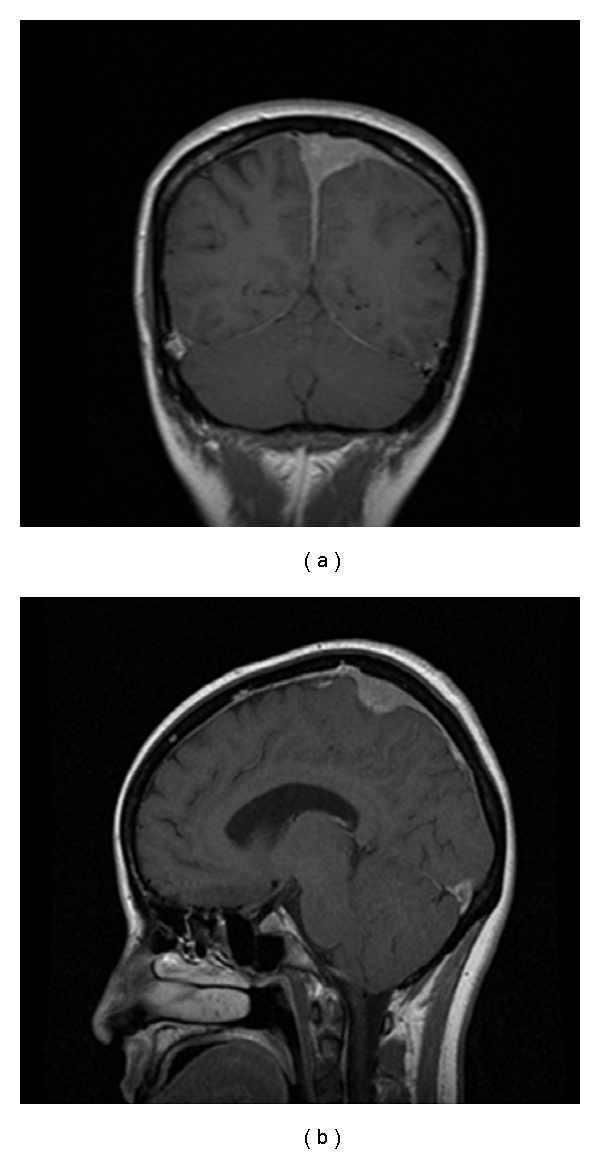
MRI at diagnosis showing broad-based attachment lesion to the meninges with indentation upon the adjacent brain parenchyma.
